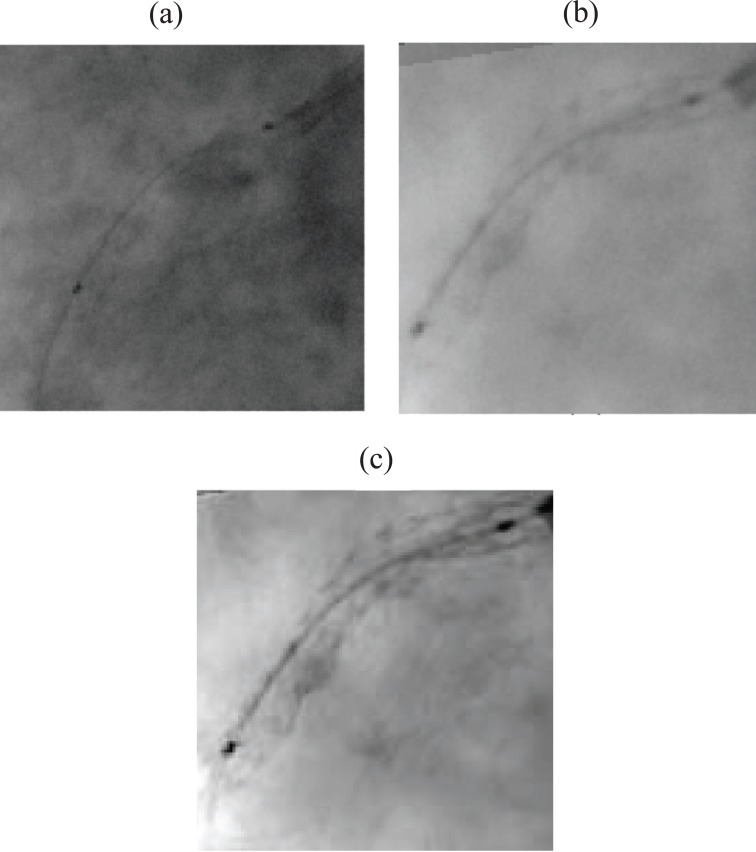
A bad quality X-ray image of coronary stent; (a), original image; (b), a crop region after StentBoost enhancement; (c), a crop region after our enhancement processing.
